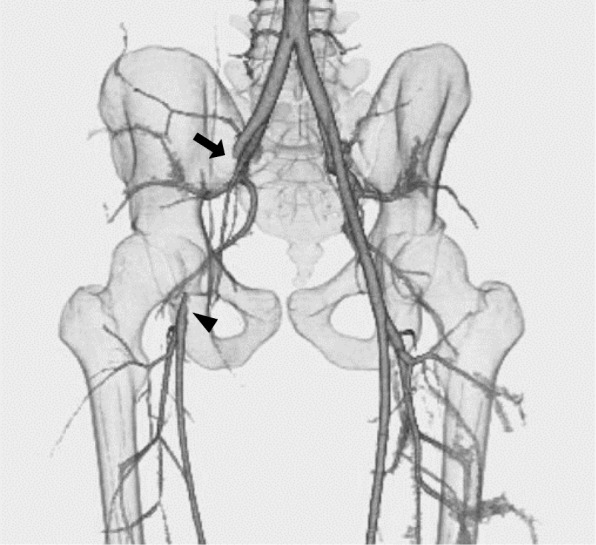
Preoperative CT angiography shows occlusion extending from the right EIA to CFA (arrow) and a contrast effect peripheral to the lesion from collateral circulation (arrowhead). CT, computed tomography; EIA, external iliac artery; CFA, common femoral artery
